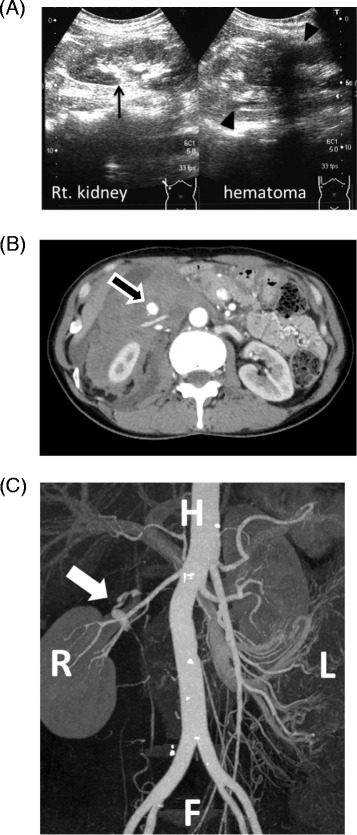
Imaging tests for retroperitoneal hematoma. (A) Abdominal ultrasonography demonstrated a normal right kidney (left side, arrow) and a large hematoma in the retroperitoneum. A high-echoic lesion can be seen surrounding the right kidney (right side, arrowhead). (B) Arterial phase contrast-enhanced computed tomography image. A bright round structure (arrow) can be seen in the right retroperitoneal hematoma. (C) Three-dimensional computed tomography angiogram of the abdomen revealed a right ovarian artery aneurysm (arrow) overriding the right renal artery. Abbreviations: L, left; R, right; Rt., right; H, head, F, foot.
